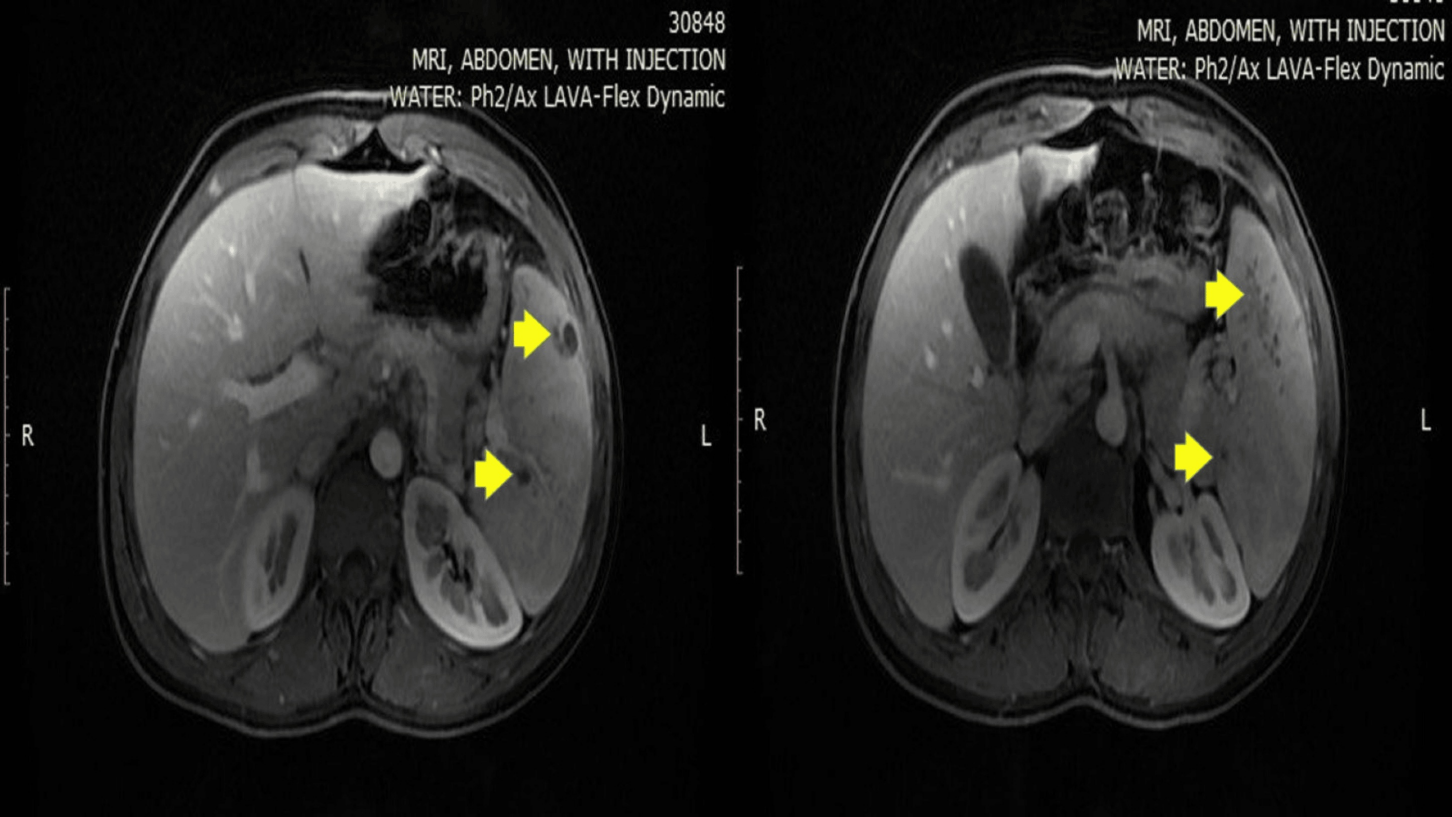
MRI abdomen with IV injection shows numerous ring enhancing lesions (yellow arrows) with imaging features, suggestive of splenic abscesses.
